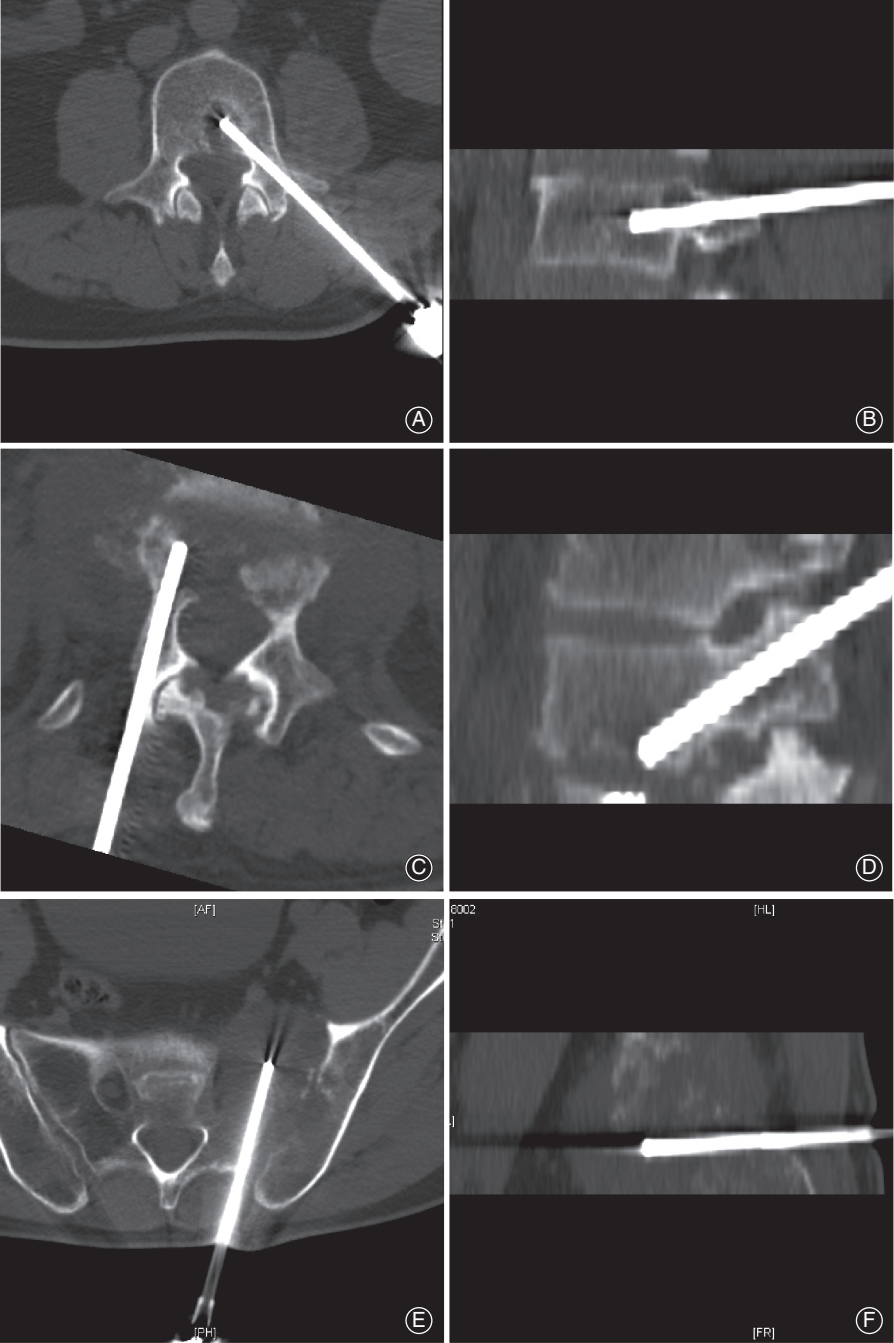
Images of CT‐guided biopsy. (A, B) The lesion is located in the posterior part of the vertebral body, close to the spinal canal. Biopsy confirmed metastatic low differentiation adenocarcinoma. (C, D) Transpedicular approach to an L_1_ lesion, located close to the inferior endplate. Biopsy showed a plasmacytosis, but a diagnosis of tuberculosis was eventually given by a multidisciplinary team. (E, F) Biopsy was performed in the left sacroiliac joint and cytology was compatible with diffuse large B‐cell lymphoma.
